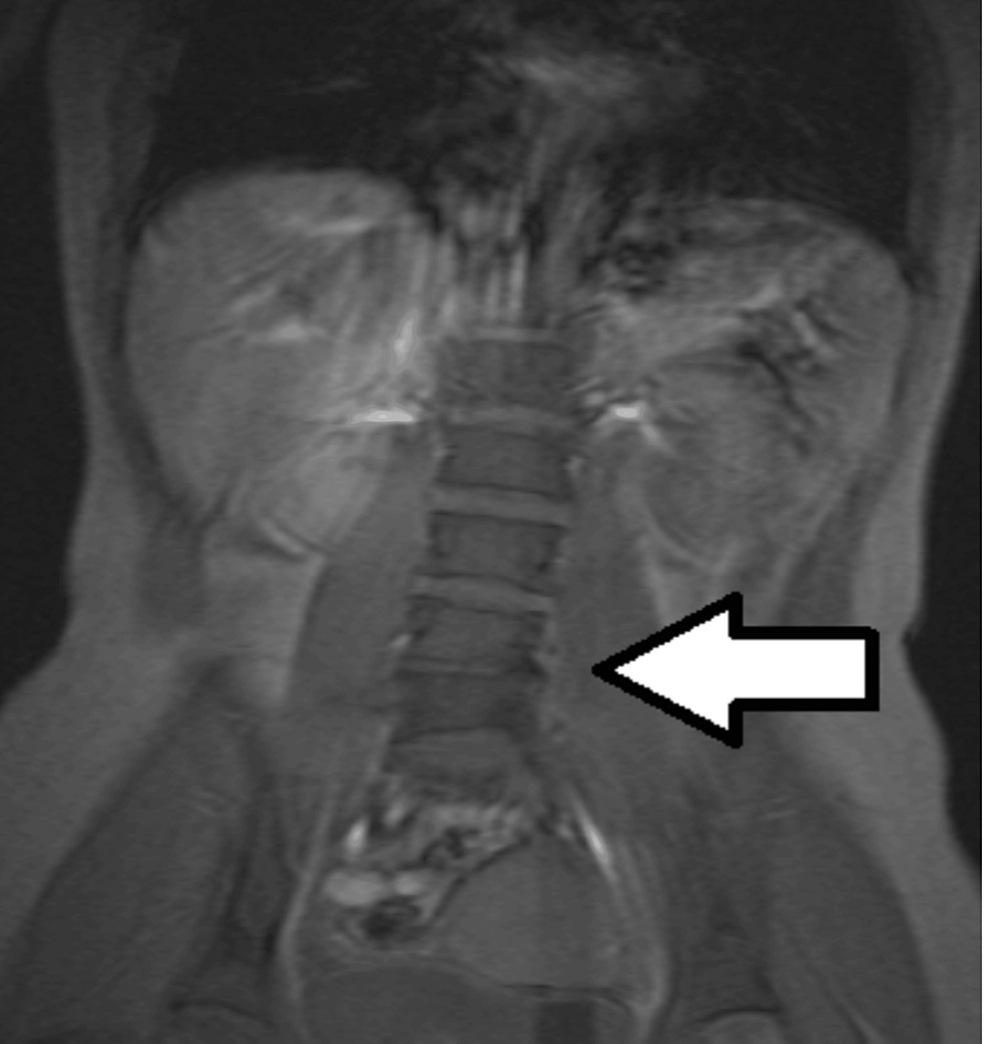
MRI of mild scoliosis in L4-L5 region Note the mild scoliosis (arrow).
